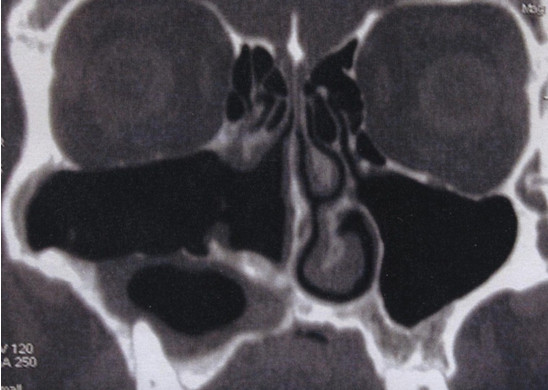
Post-operative computed tomography scan taken at the 8-year follow-up examination. Scan shows no keratocystic odontogenic tumor recurrence to the right maxillary sinus and scarce pathologic tissue in the alveolar recess of the right maxillary sinus.
